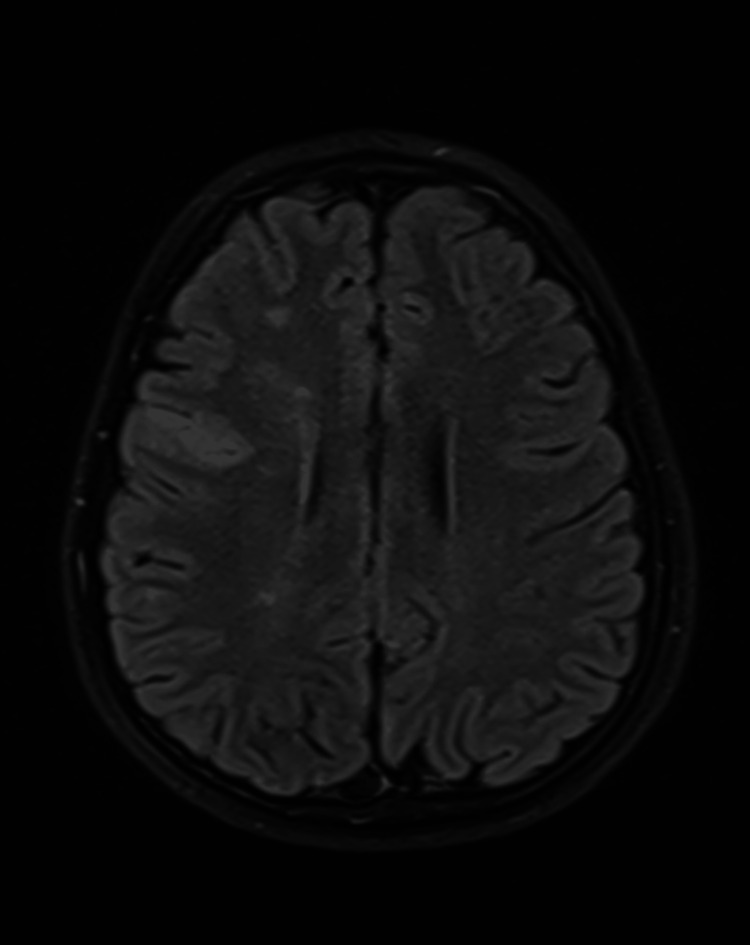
MRI, Axial FLAIR image showing abnormal high signal in the right frontal lobe cortex and also in the centrum semiovale in the deep watershed area.
